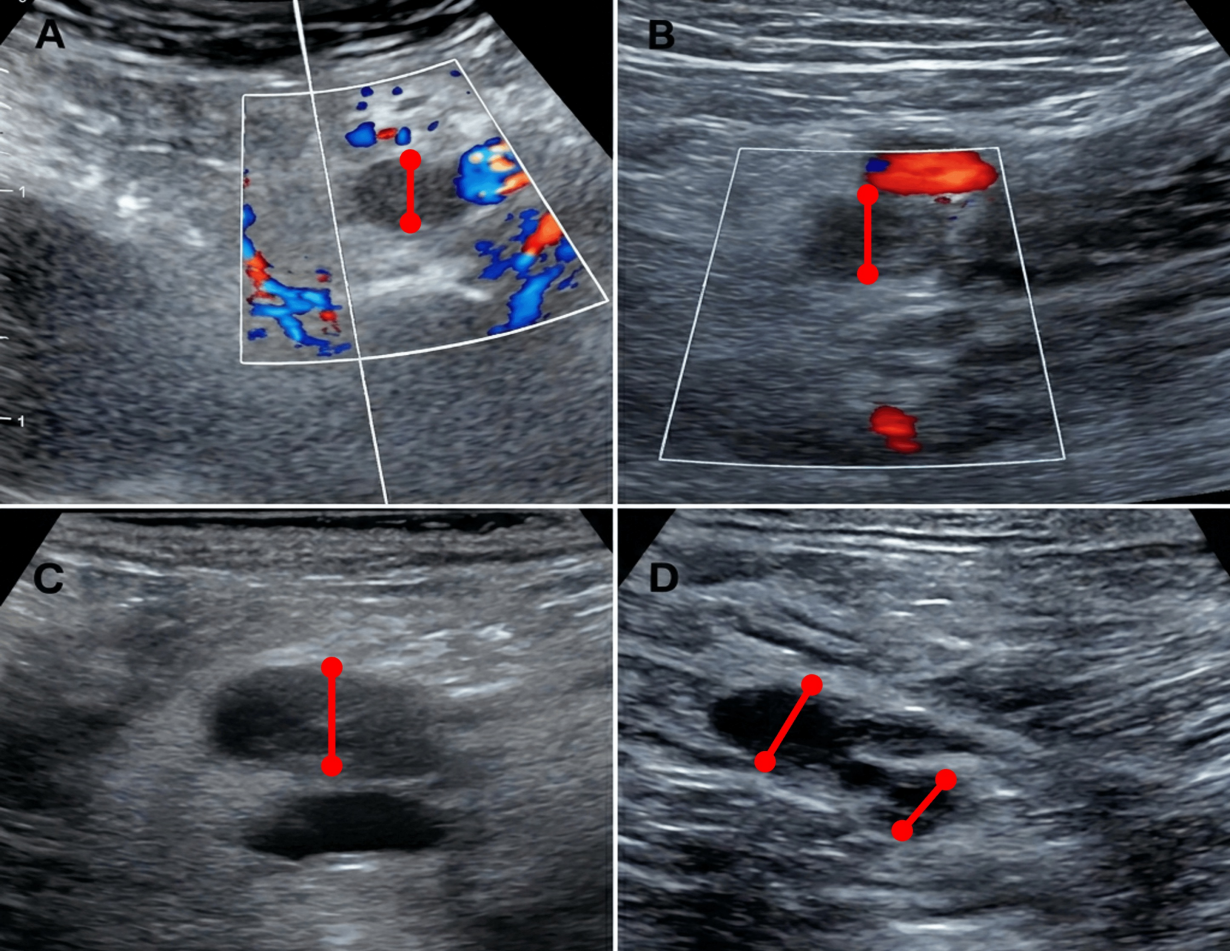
Venous Doppler ultrasound demonstrating extensive thrombosis of the left lower limb. The measurements represent the maximal venous diameter in the affected non-compressible segments: left external iliac vein (15 mm; A), common femoral vein (12 mm; B), popliteal vein (11 mm; C), and posterior tibial veins (7 mm on the left and 5 mm on the right; D).
